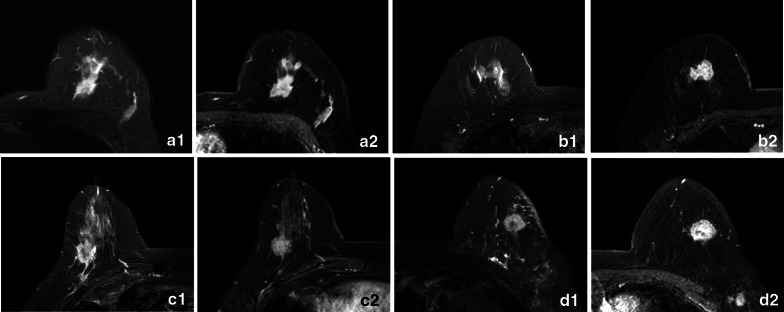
Examples of breast edema score on MRI. a A 43-year-old female presented with pathologically confirmed left breast cancer without axillary lymph node metastasis. IHC: ER (80% +), PR (90% +), KI67 (10% +), HER-2 (−). Axial fat-suppressed T2 weighted image (T2WI) showed no high signal intensity (no edema, BES 1) in the breast. Axial post-contrast T1 weighted image (DCE-MRI) showed the spiculated mass heterogeneously enhanced. b A 68-year-old female presented with pathologically confirmed right breast cancer with eight axillary lymph nodes metastasis. IHC: ER (−), PR (−), Ki-67 (30% +), HER-2 (−). T2WI showed the signal intensity around the tumor mass was as high as that of water (peritumoral edema, BES 2). DCE-MRI showed the apparent enhancement of lesion. c A 69-year-old female presented with pathologically confirmed right breast cancer with four axillary lymph nodes metastasis and lymphovascular invasion. IHC: ER (90% +), PR (70% +), KI67 (30% +), HER-2 (−). T2WI showed a line-shaped hyperintensity extended to the anterior space of pectoralis major muscle (prepectoral edema, BES 3) and high intensity around the mass. DCE-MRI showed the obvious enhancement of lesion. d A 64-year-old female presented with pathologically confirmed left breast cancer with fourteen axillary lymph nodes metastasis. IHC: ER (−), PR (−), KI67 (30% +), HER-2 ( +). T2WI showed the skin adjacent to the mass thickening and the hyperintensity of skin and subcutaneous fat (subcutaneous edema, BES 4) and high intensity in the prepectoral area. DCE-MRI showed the oval mass heterogeneously enhanced
